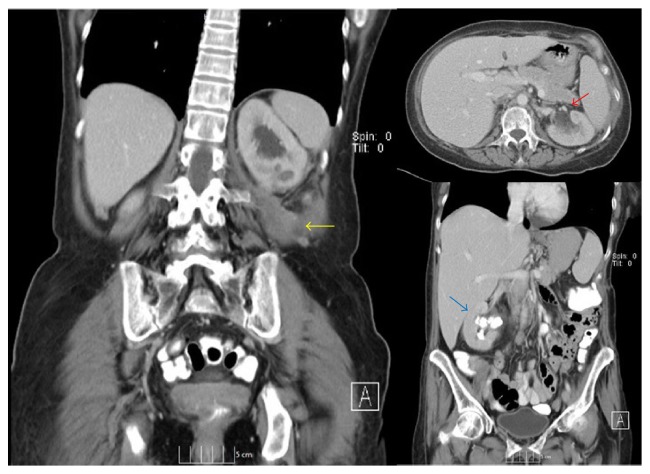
Postoperative CT scan performed 2 months after surgery showed a small residual retroperitoneal collection measuring 2.1 × 1.0 cm (Yellow arrow) and interval resolution of left upper pole hydronephrosis (red arrow) and a right kidney with a nonobstructive large UPJ calculus (blue arrow).
